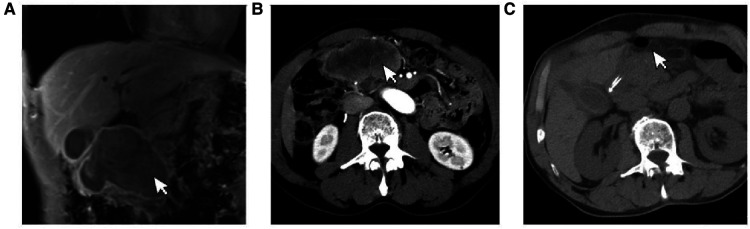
(A) The gastric antrum occupies space, the main lesion is located outside the gastric wall. (B) Patient's preoperative CT showed obvious lesions. (C) Postoperative CT of the patient showed significant resection of the lesion.
